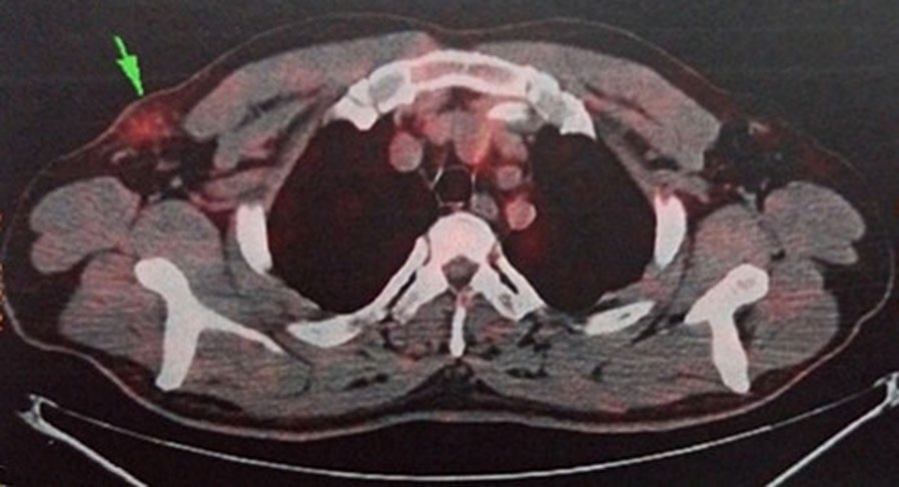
The PET–CT scan: axillary lymph nodes (*arrow*). Radioactive tracer: 18F-FDG
